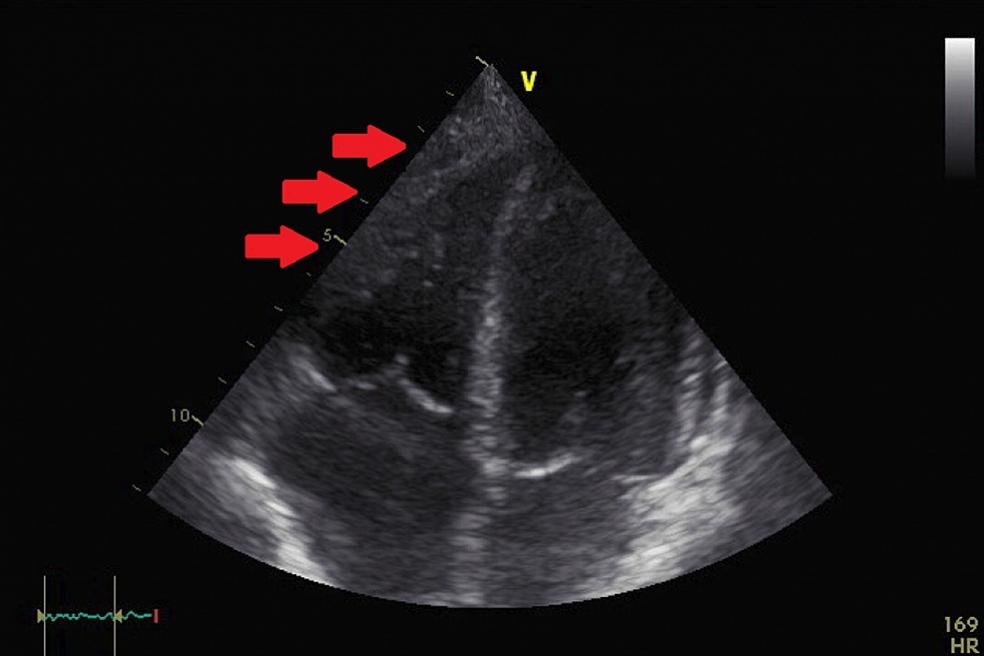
Apical four chambers view of the heart showing absence of the pericardium on the right ventricle area (red arrows).
